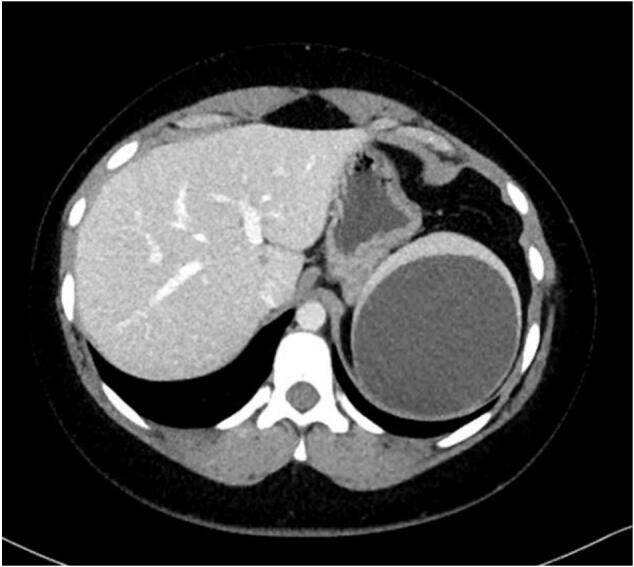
Axial view of the splenic cyst on CS CT scan.
